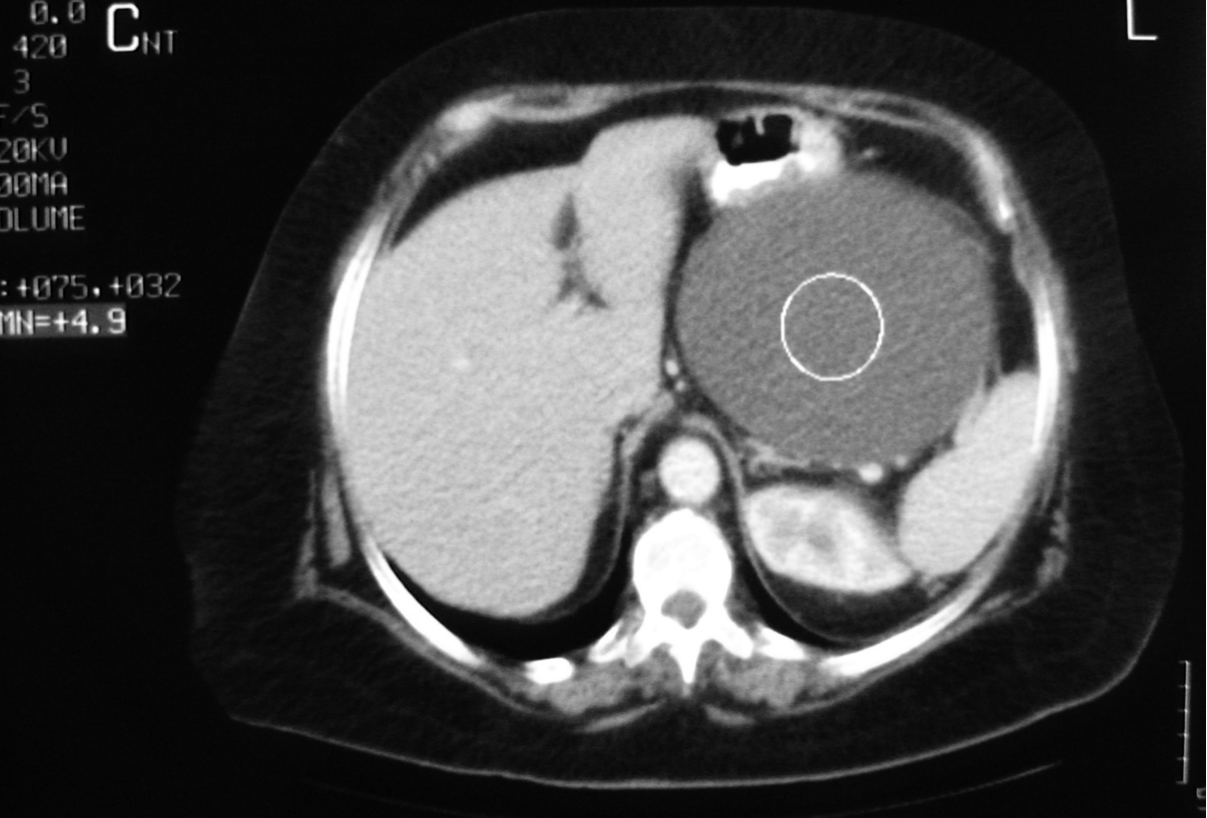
CT examination with the Hounsfield (HU) value of the lesion demonstrating its cystic nature.
